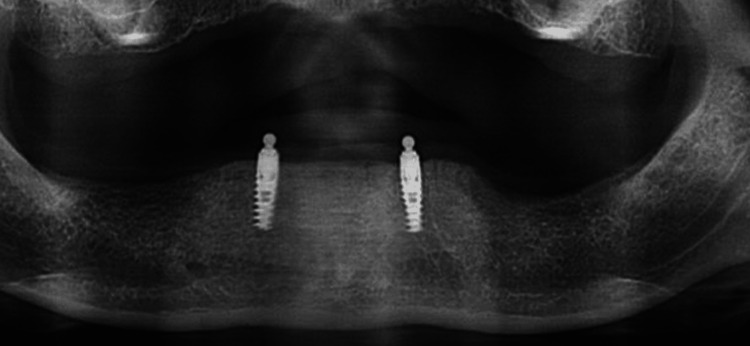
Radiograph after 3 years.
